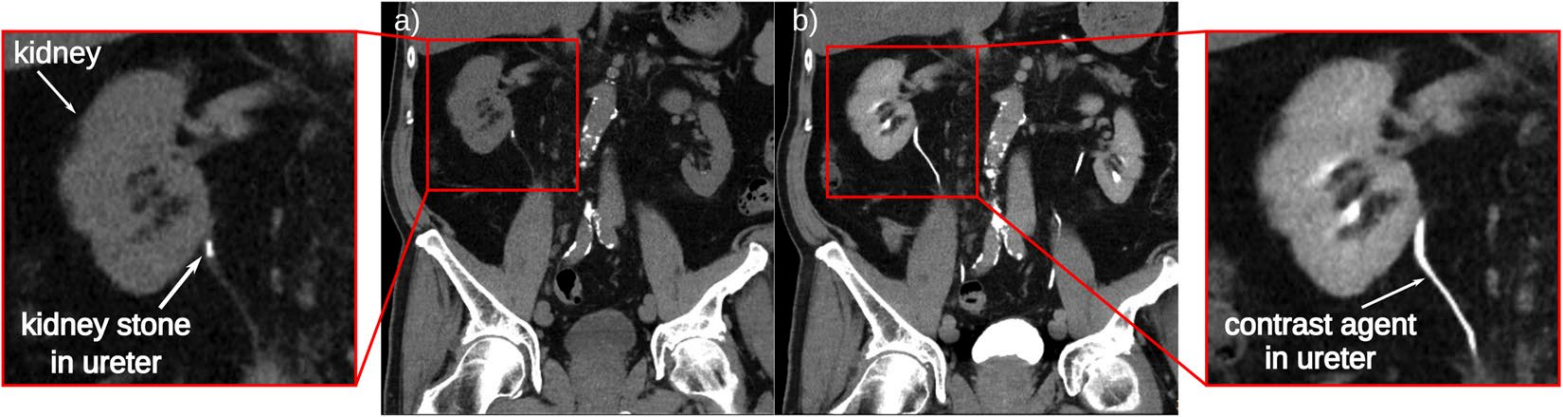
Clinical X-ray CT images before (a) and after (b) administration of iodine contrast agent. In the CT image in (a) the kidney stone is clearly visible in the proximal part of the right ureter. However, after the injection of contrast agent (b) the kidney stone in the ureter cannot be distinguished from the iodine contrast agent and would be missed.
